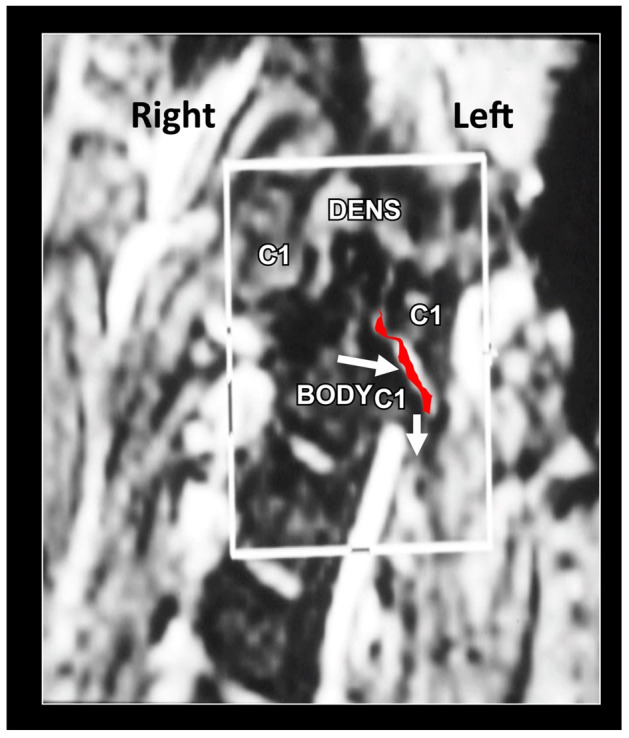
Atlas*-*axis* gapping. Left coronal, dynamic magnetic resonance imaging (MRI), showing the lateral gapping of the left facet joint between the *atlas* and *axis*, during lateral cranial rotation (side bending) restriction. The lack of rotational component of the *axis* during lateral rotation of the cranium to the same side induces a dysfunctional pattern of cranial rotation. When the *axis* attempts to rotate towards one side, the superior lateral facet joint of *axis* drops down on the same side of the lateral rotation of the cranium, opening the gap between the lateral mass of the *axis* below the *atlas*. The lateral evaluation of the *axis* is painful on the restricted side of the lateral movement. The *axis* cannot rotate to that side and descends below the *atlas* causing capsular distension of the atlanto-axial joint. The MRI facilitates the understanding of the dysfunction to plan the treatment process and left rotation of the *axis* on the C3 vertebra. During treatment, it is important to maintain proportional rotational patterns of movement between *atlas* and *axis*.
